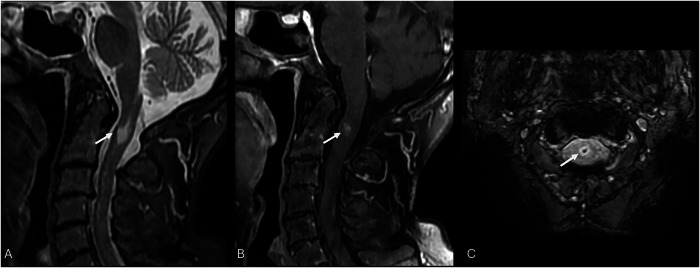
Cervical spine MRI scans show an intradural mass at the C2 level. The mass shows high-signal intensity on sagittal T2-W FS STIR image (white arrow, A), homogeneous and strong enhancement (white arrow, B), and blooming artifacts on axial SWI (white arrow, C). These are incidental findings of a rare spinal cord capillary telangiectasia
